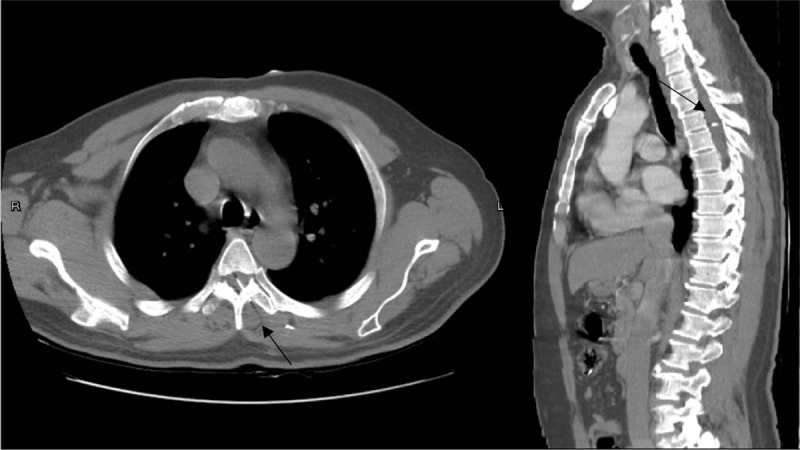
Computed tomography scan showing entrance of the nail through the left T5-6 spinal canal and soft tissue of the left back.
